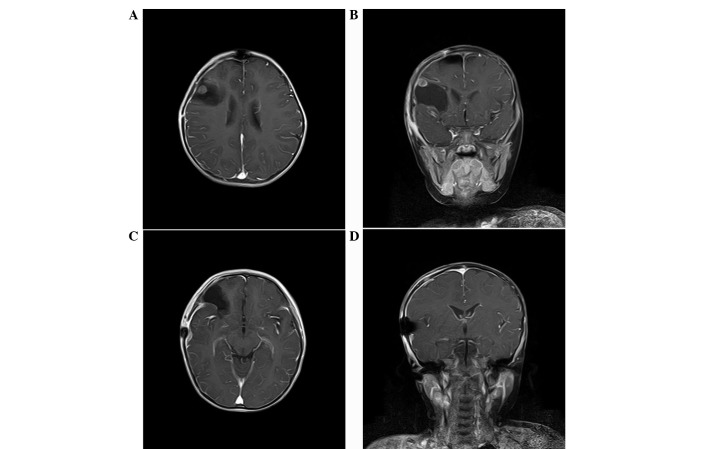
MRI scan with gadolinium diethylenetriamine pentaacetic acid administration was performed one month after the initial resection. (A) A solid node in the initial surgery field was identified and (B) a coronal image was captured. (C and D) Enhancement of the other intradural tumor node was identified at the posterior edge of the bone window. MRI, magnetic resonance imaging.
